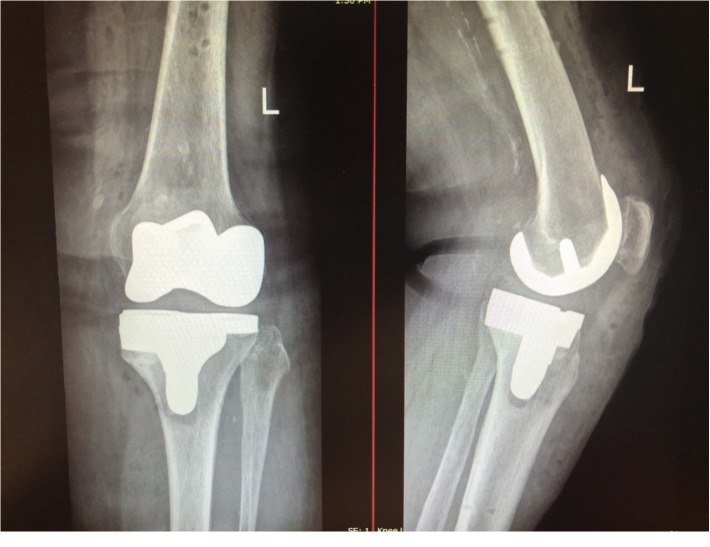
Postoperative anteroposterior and lateral X-ray views.
